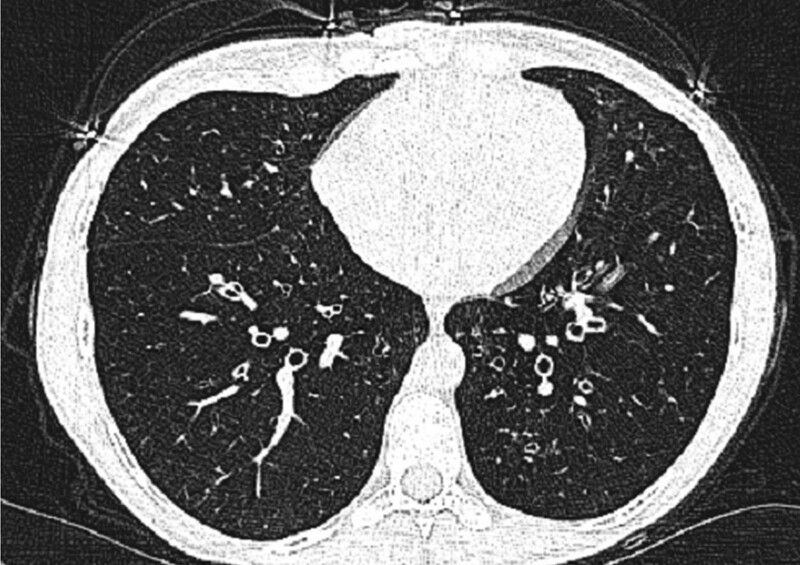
Chest computed tomography revealed bilateral bronchiectasis.
